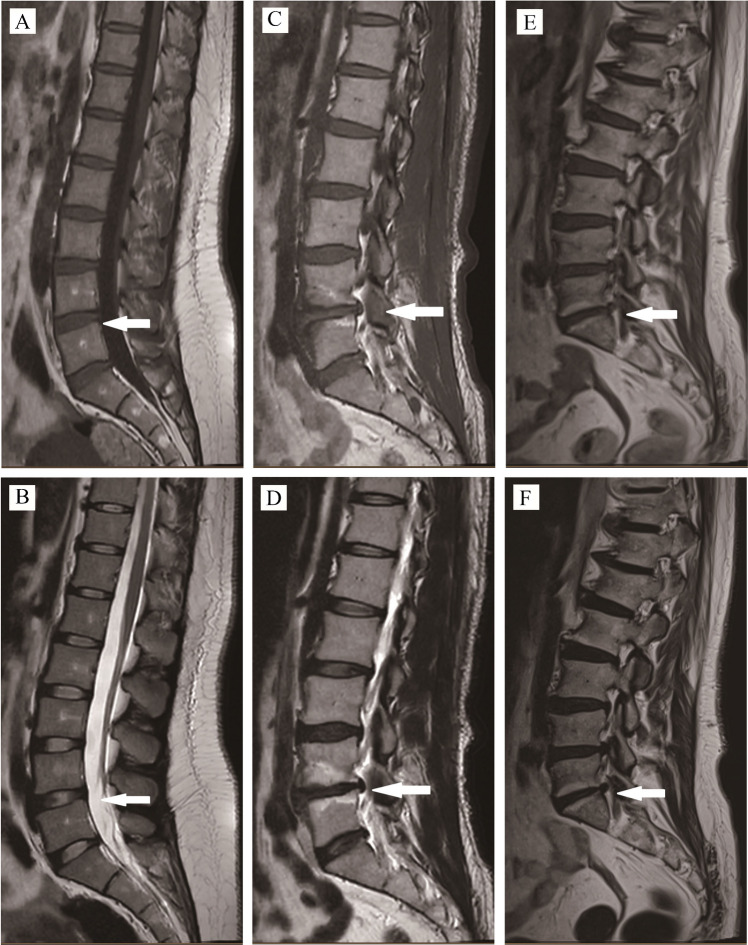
Appearance of Modic changes in intervertebral discs. (A, B) Normal discs; (C, D) Type I, Hypointensity onT1-w and hyperintensity onT2-w. (E, F) Type II, hyperintensity on T1-w and T2-w (White arrow). MRI, Magnetic resonance imaging; -w, weighted imaging
